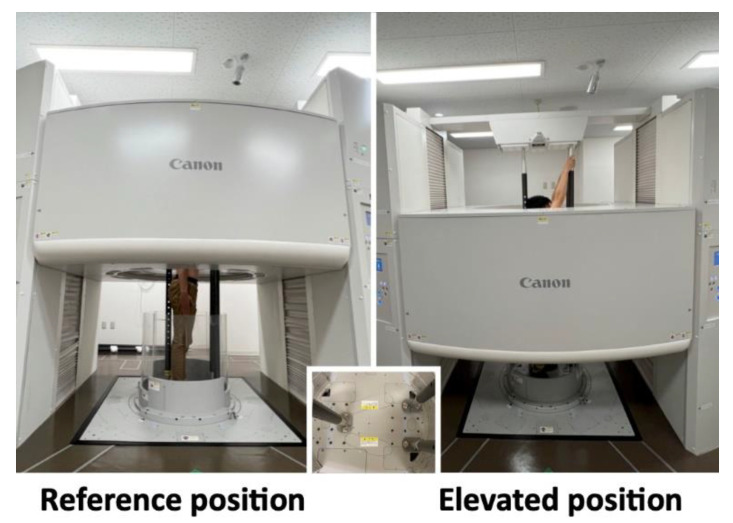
Upright computed tomography. To keep the reference and elevated positions during the scanning, three vertical poles were installed in the gantry.
